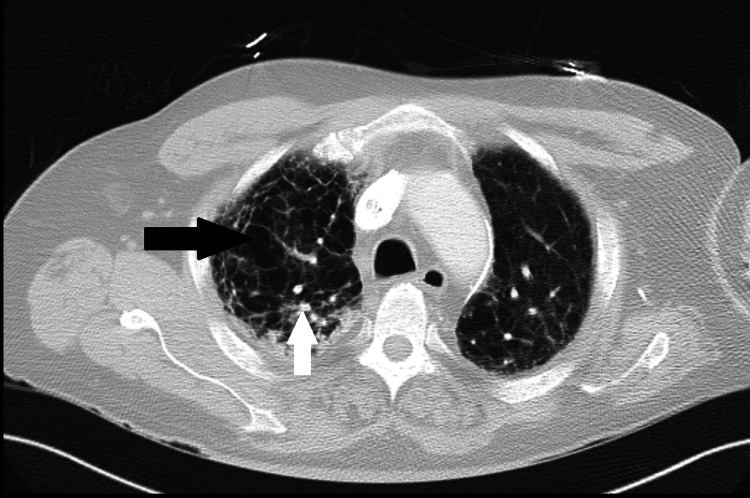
Computed tomography of the upper-middle lung Upper-middle lung CT shows diffuse emphysematous changes (black arrow) with a bullous disease, as well as calcified nodules (white arrow).
